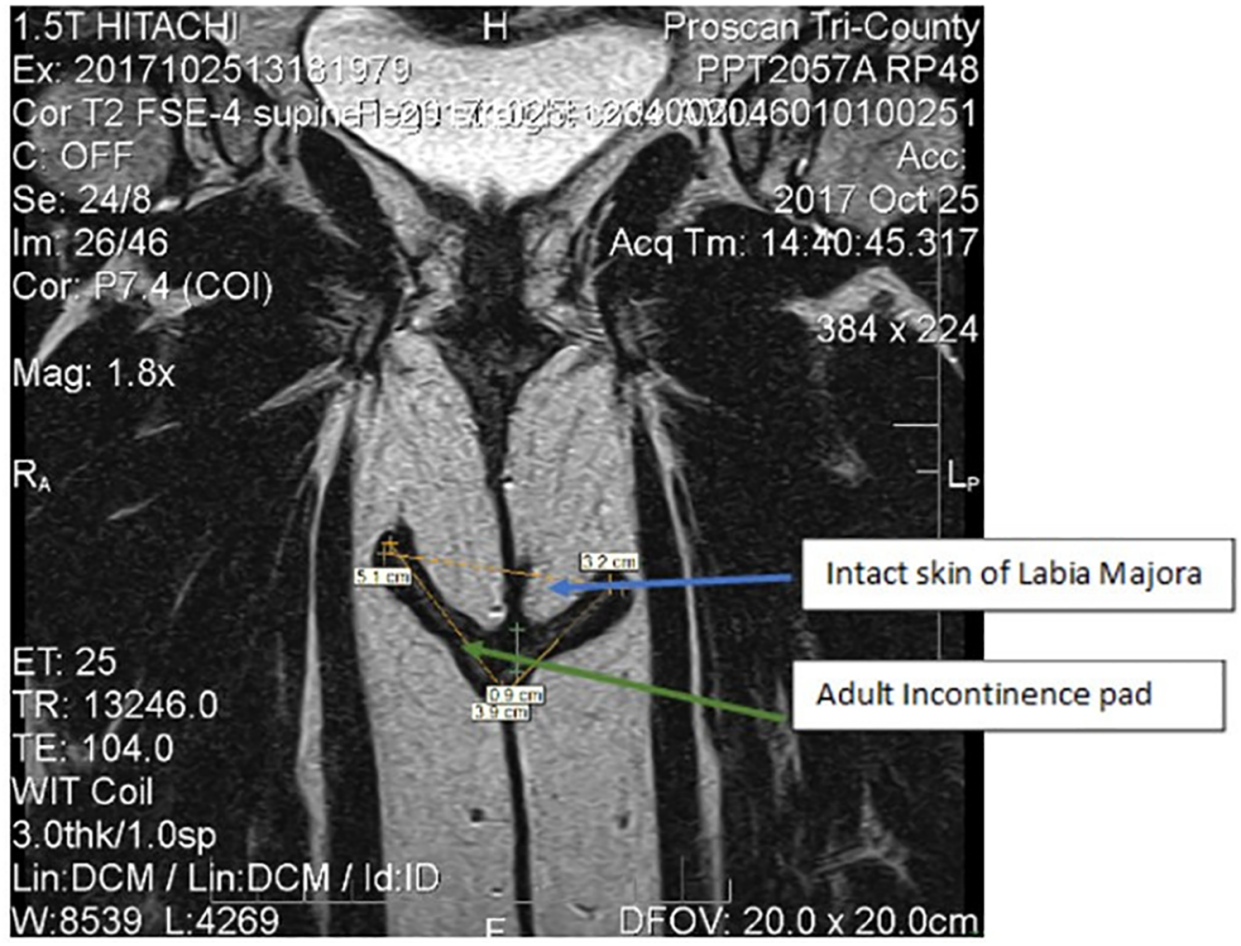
Frontal plan images via magnetic resonance imaging of female anatomy of the lower urogynecological region. The yellow line shows the orientation of the pad in contact with external structures of the pad (green arrow). The blue arrow shows the structures of the labia majora.
